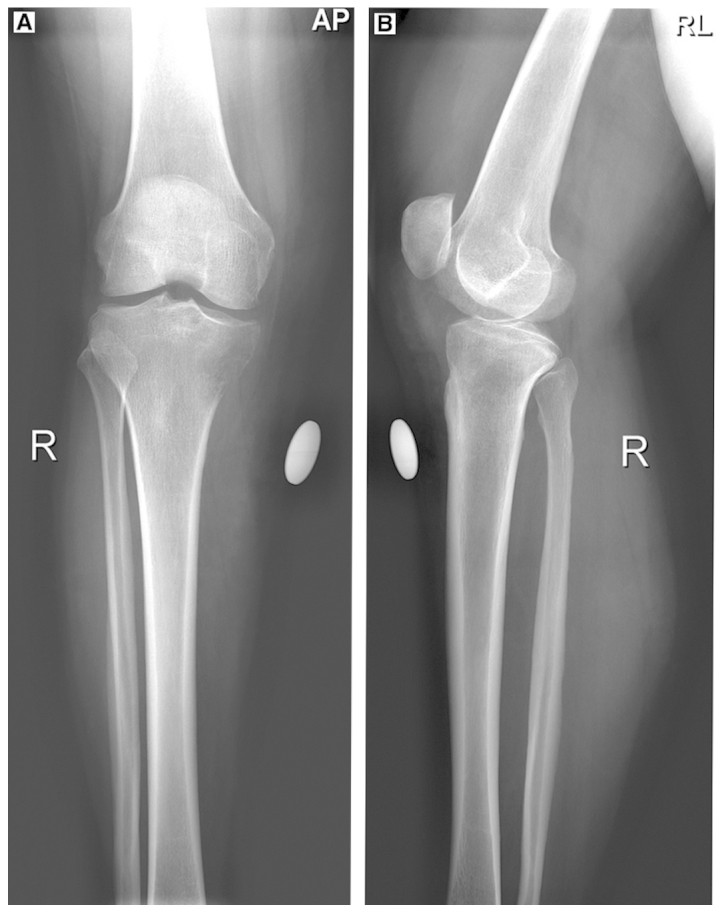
X-ray scan revealed the presence of a space-occupying lesion in the right knee medial tibia platform. (A) Posteroanterior examination and (B) lateral examination.
